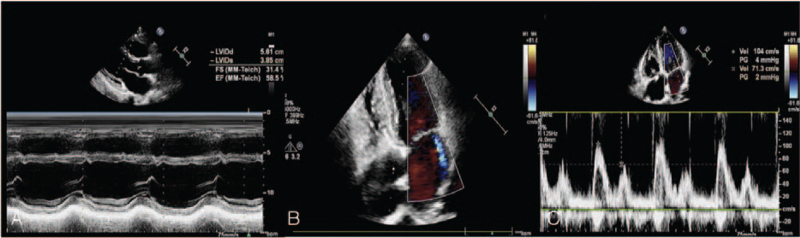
Echocardiographic imaging of this patient after operation. (A) M mode and two-dimensional echocardiography showed cardiac enlargement with no mass, and the LVEF was 59%. (B) Color doppler imaging showed mild mitral regurgitation; (C) Pulsed Doppler showed the normal forward flow velocity of mitral valve.
